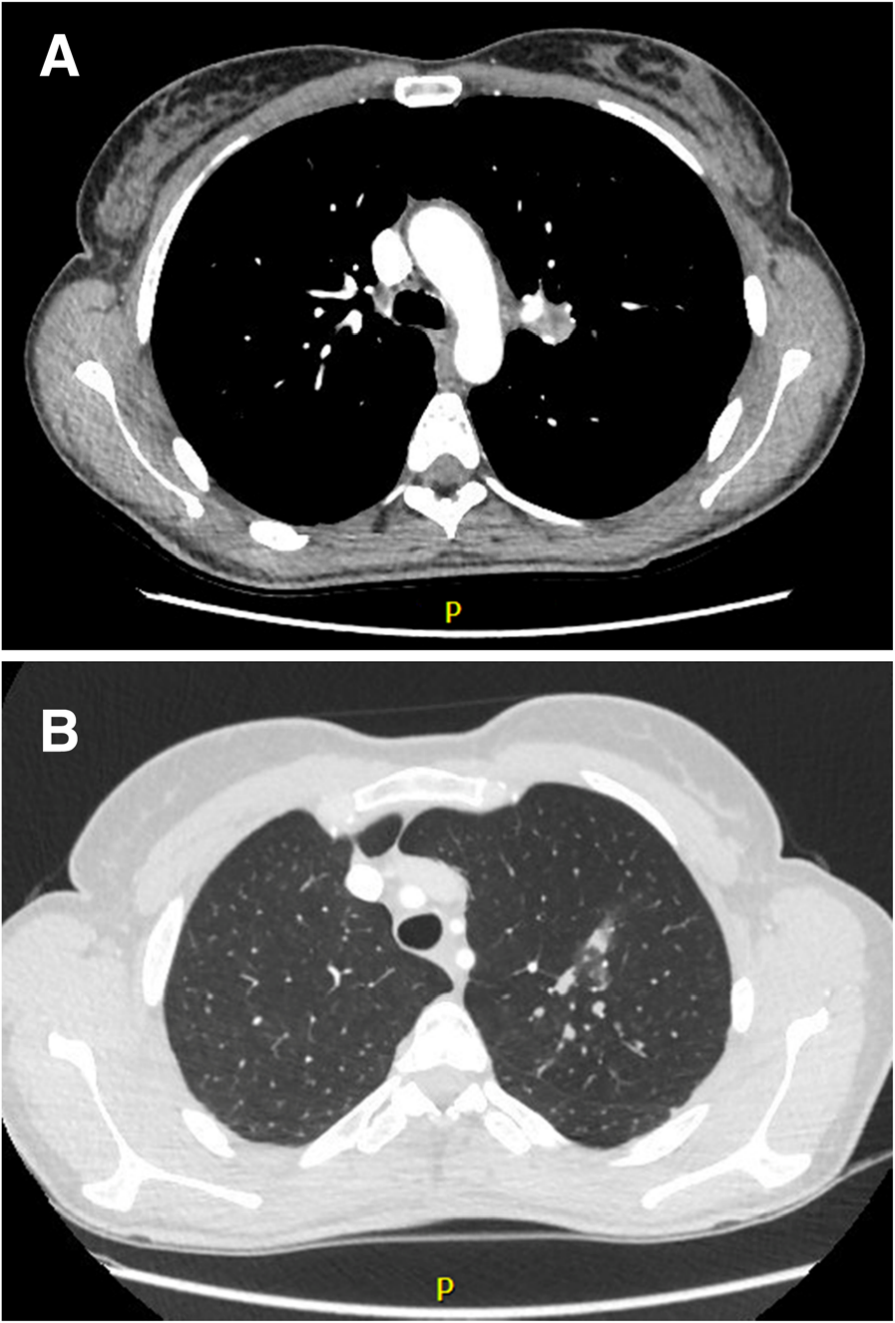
Computed tomography (CT) of the thorax showing the bronchial lesion in the left upper lobe (a) and a tumor mass at the location of the left upper lobe bronchus and some consolidation in the lung parenchyma of the left upper lobe (b)
